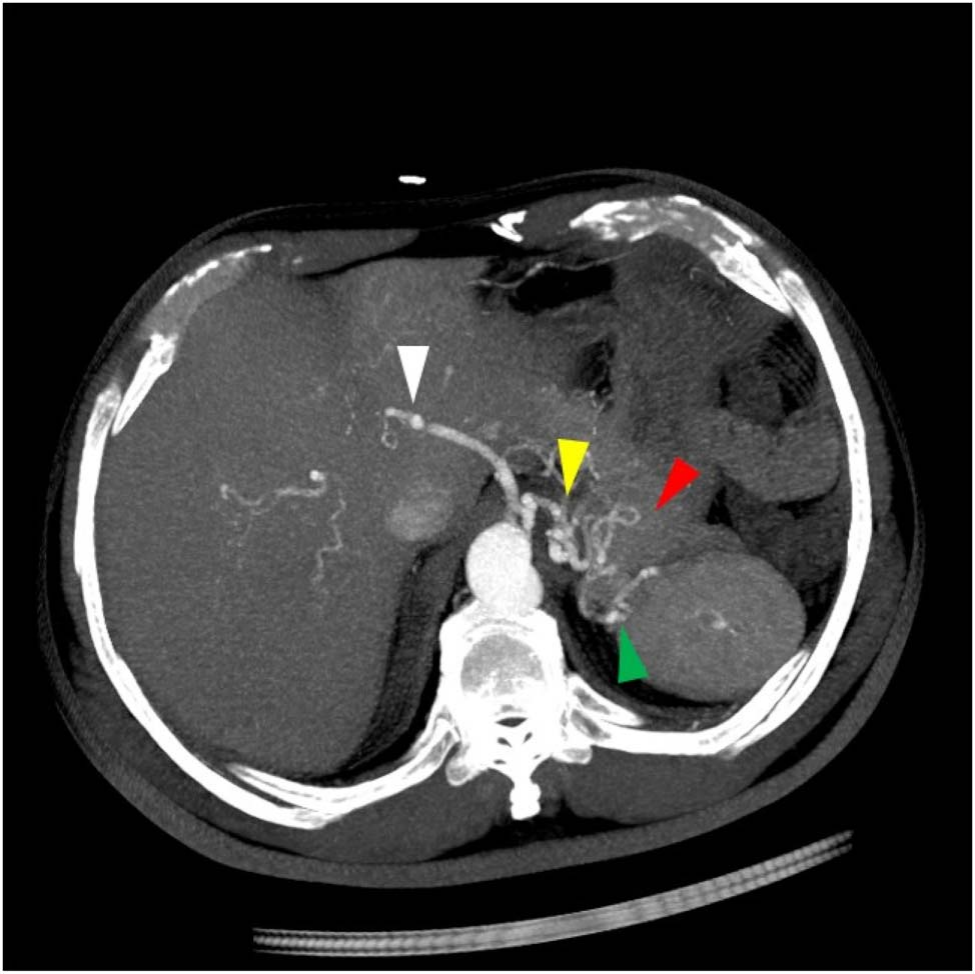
Oblique maximum intensity projection computed tomography image showing the course of the aberrant vessel, including the left inferior phrenic artery (yellow arrow) branch coursing into the stomach (red arrow) and to the splenule (green arrow). The celiac artery (white arrow) has no splenic branches.
